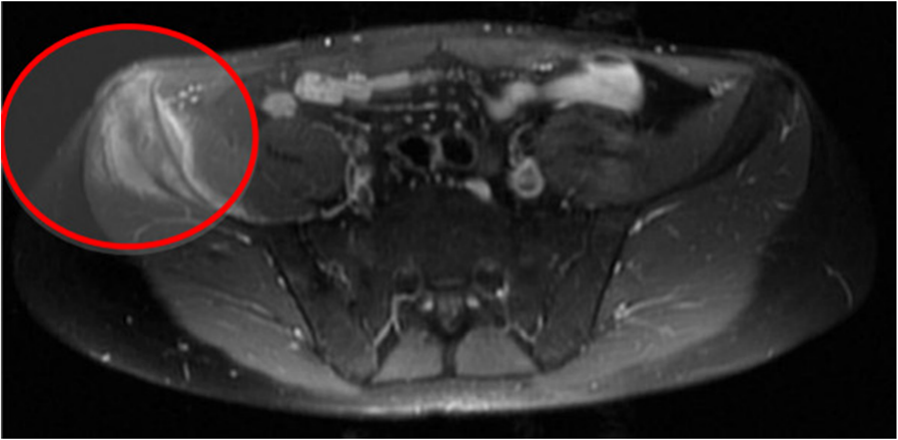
Axial MRI showing the involvement of the right iliac crest.
